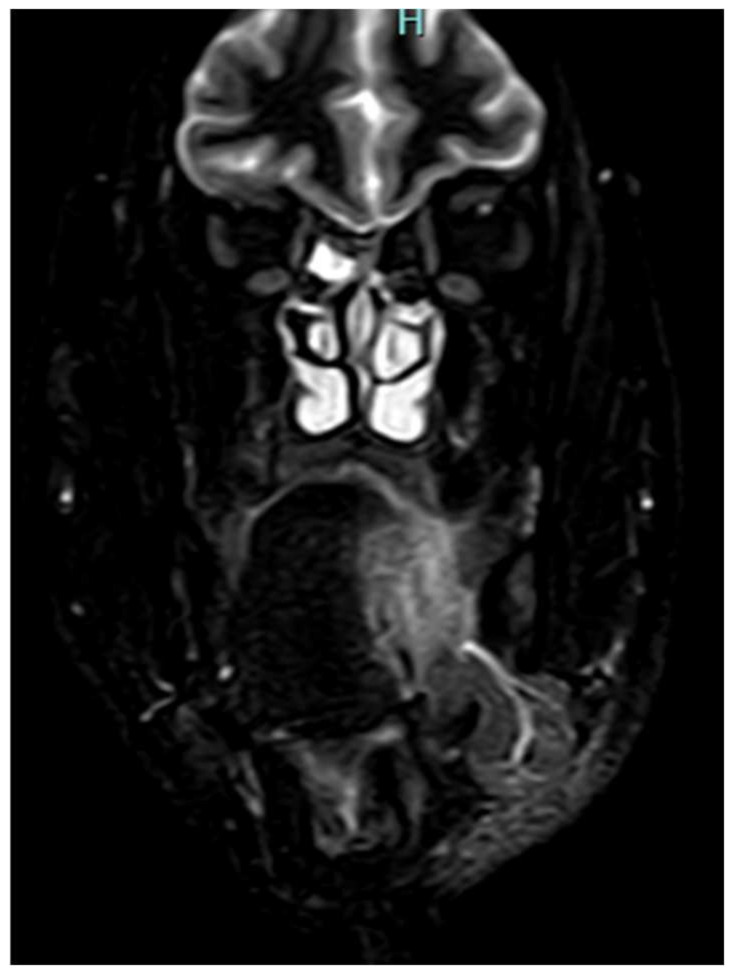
Coronal magnetic resonance imaging (MRI), demonstrating a key diagnostic finding of lateral extension of the primary lesion into the tonsil and tonsillo-lingual sulcus, resulting in a confluent mass involving both the tumor and an ipsilateral N3b nodal metastasis (node–primary complex).
